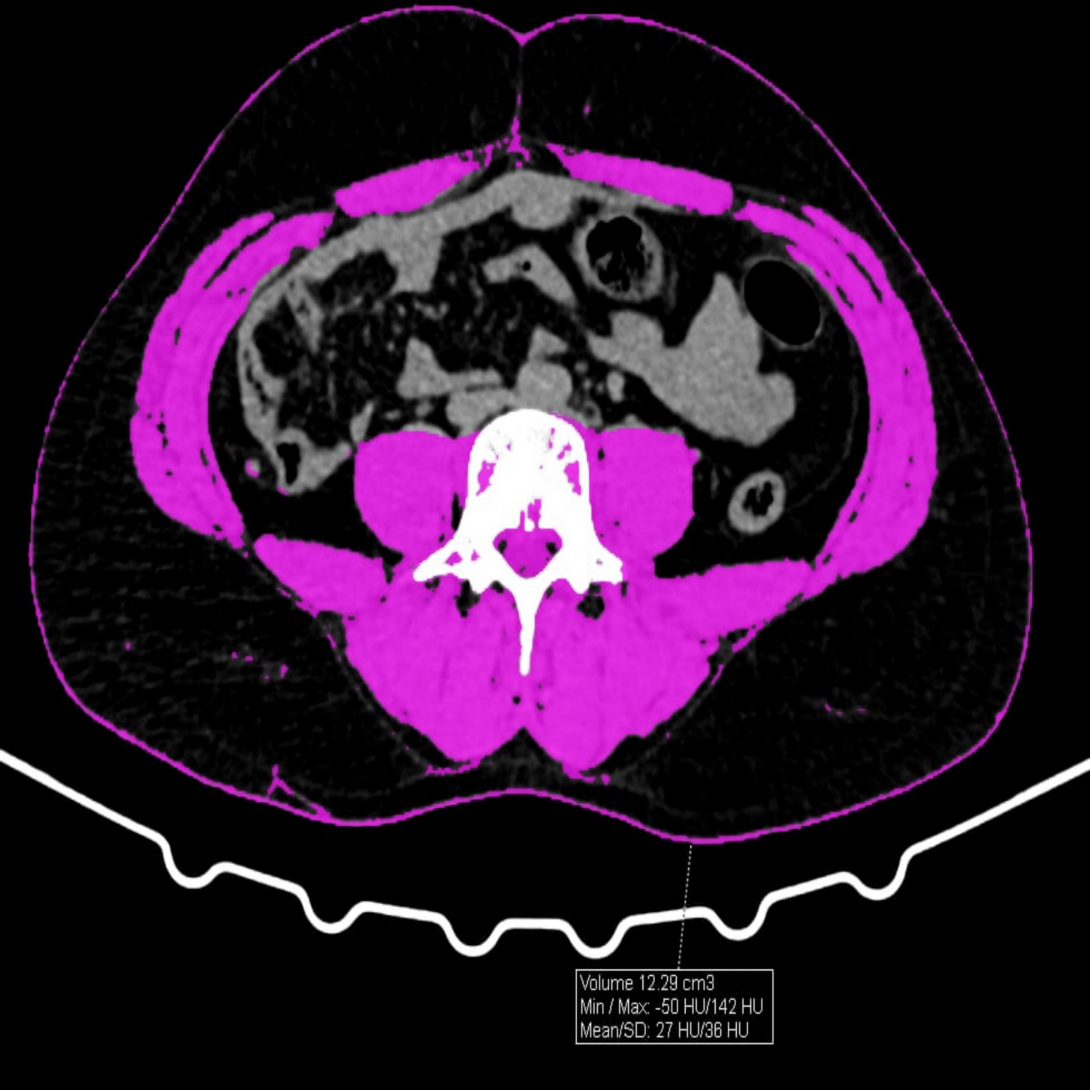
SMT calculations by CT within the ROI (region of interest) SMT: Skeletal Muscle Tissue
